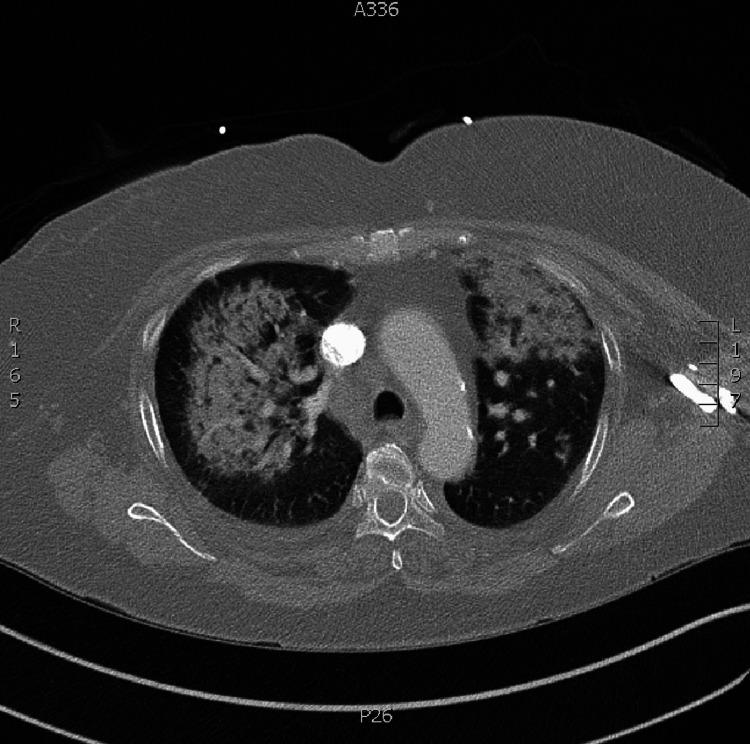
Chest computed tomography scan (lung window) during the primary admission showing bilateral patchy pulmonary infiltrates/consolidations, air bronchograms, bilateral ground glass opacities, and a small right-sided pleural effusion.
